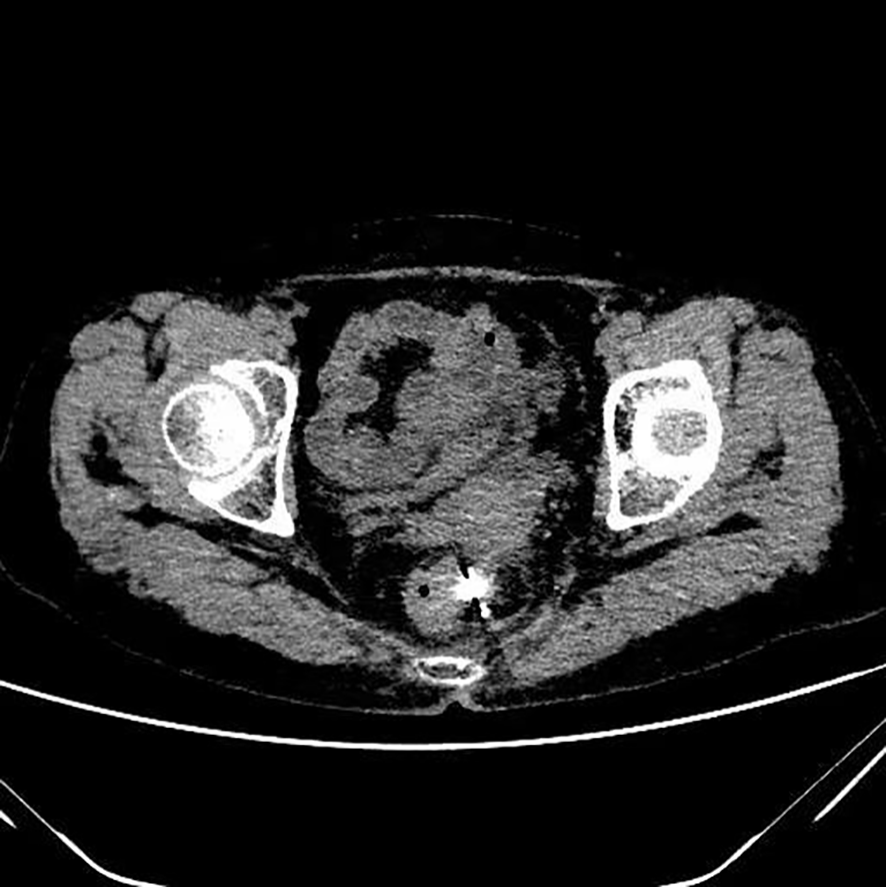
Postoperative pelvic CT image of the patient showing a solid mass in the recto-uterine pouch, with hyperdense radioactive seed implants visible within the lesion.
